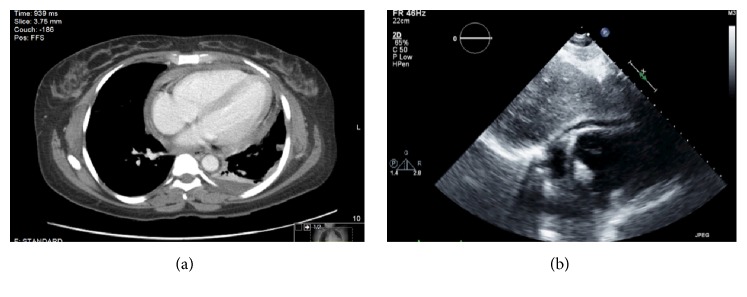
(a) Small pericardial effusion. Subsegmental atelectasis at the left lower lobe. Small left pleural effusion; previous right pleural effusion has resolved. (b) Parasternal long-axis echocardiogram showed small pericardial effusion with no evidence of echo cardiac tamponade.
